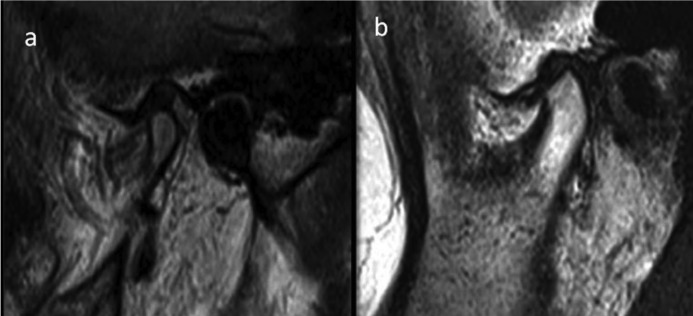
a): MRI of control showing normal appearance of condylar head. (b): MRI of OSMF patient showing flattening of condylar head.
